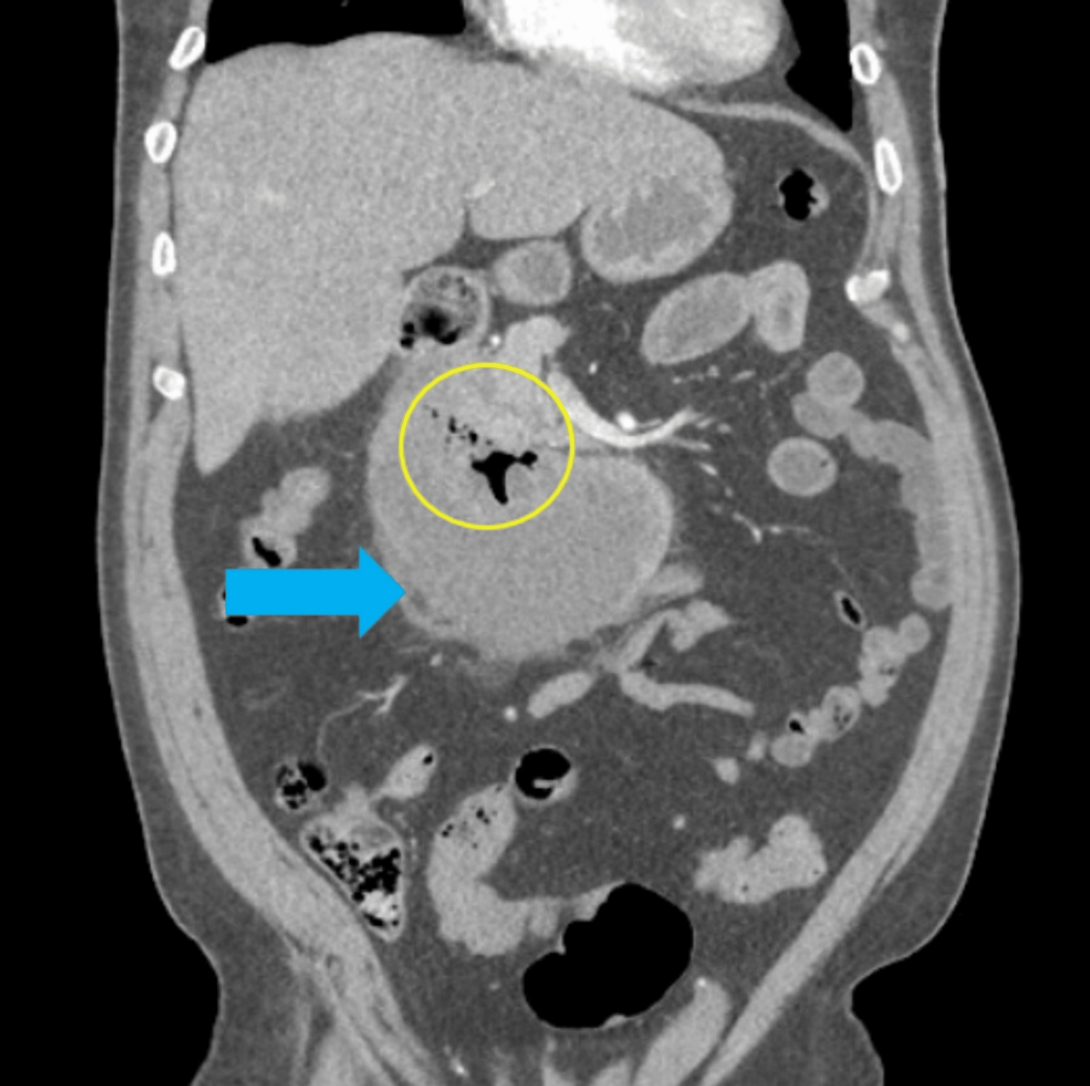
Computed tomography angiography (CTA) of the abdomen and pelvis, coronal view, lung window. Imaging performed 16 hours after endoscopic evaluation demonstrated an enlargement of the duodenal hematoma (blue arrow) in addition to a new cluster of gas bubbles (yellow circle).
